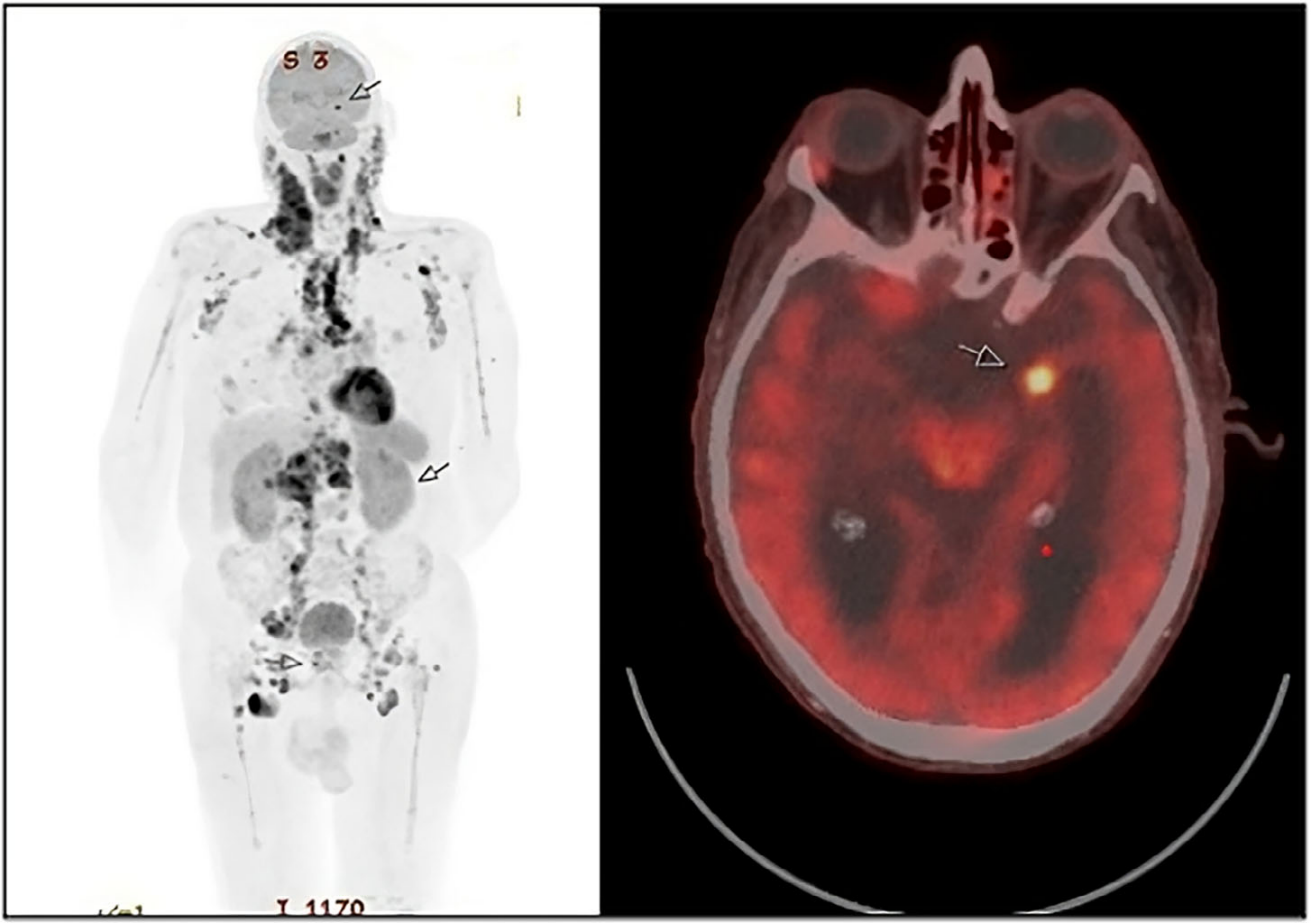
Positron emission tomography (PET) at diagnosis. This shows metabolically active lymphoma above and below the diaphragm with a focal increase in fluorodeoxyglucose (FDG)‐avidity in the medial aspect of the left temporal lobe with a standardized uptake value (SUV) max of 8.4 consistent with central nervous system (CNS) involvement by mantle cell lymphoma (MCL).
